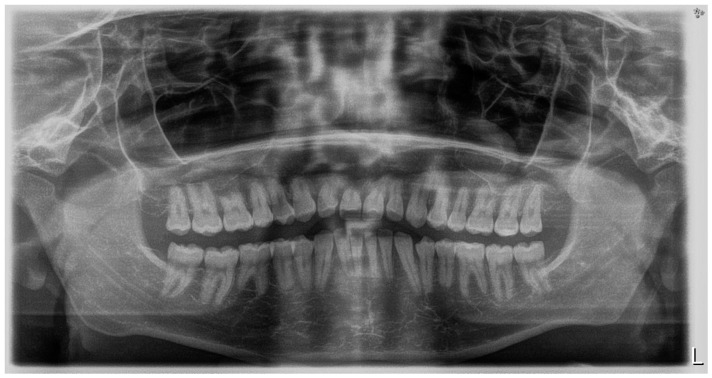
Panoramic radiograph one year after debonding.
